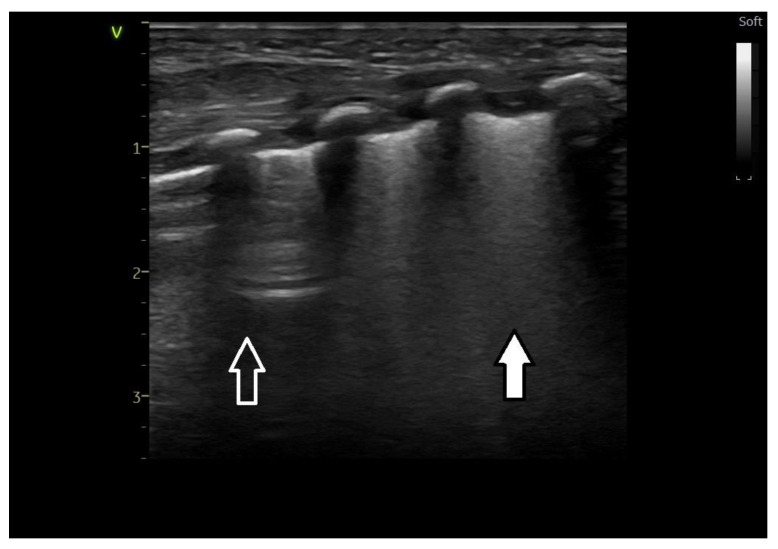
Lung ultrasound pattern in transient tachypnea of the newborn—double lung point—type A and type B lines visible in the upper lung fields—arrow with white outline; white lung appearance in the lower lung fields—white arrow. Compare with Figure 1—normal lung and Figure 2—lung ultrasound appearance in respiratory distress syndrome.
